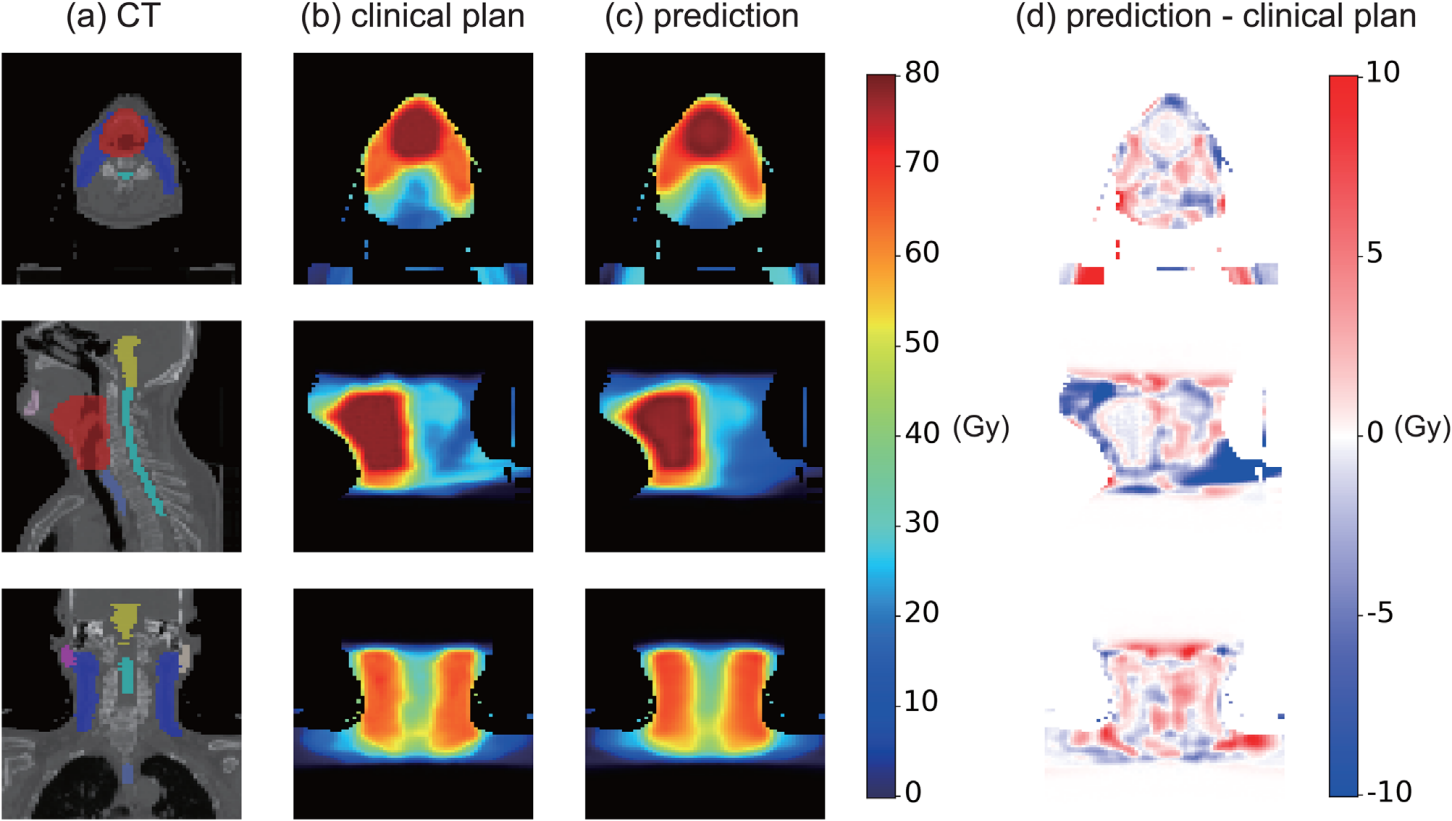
Comparison of the clinical and TrDosePred ensemble predicted dose maps for a patient in the test dataset. (a) CT, (b) clinical dose, (c) the prediction of the TrDosePred ensemble, (d) the difference between the prediction and clinical dose (prediction ‐ clinical).
